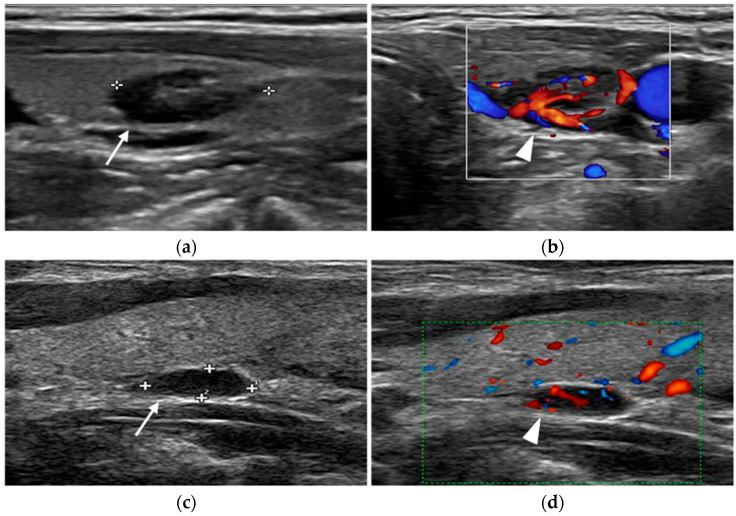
Parathyroid adenomas. In two different patients, ultrasound shows a markedly hypoechoic nodule (arrow) close to the left lobe (a) and right (c) thyroid lobe. Color-doppler images highlight the feeding vessels (arrowhead) inside the lesions (b,d).
